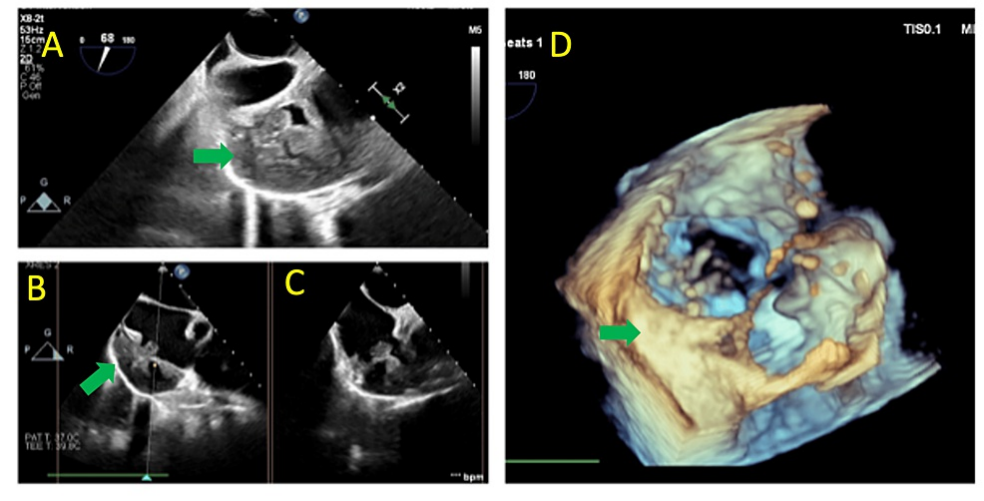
2D TEE (A,B,C) and 3D TEE imaging (D) showing an isoechoic lobulated structure (Green arrow) in the right atrium just above the tricuspid valve suggestive of a mass/thrombus. It measures 4.8cm x3.7cm. In the mass/thrombi there is a hypoechoic avascular structure indicative of cyst or fluid collection measuring 2.3cm x1.1cm. A loculated moderate pericardial effusion is noted measuring 1-2 cm. TEE: Transesophageal echocardiogram Green Arrow: right atrium mass
